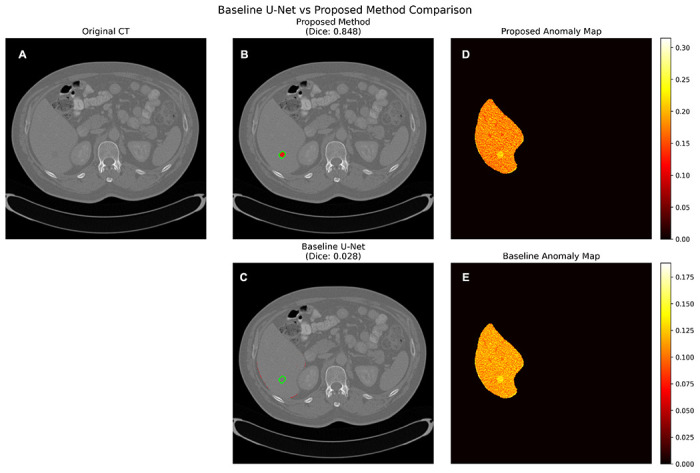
Baseline U-Net comparison. Side-by-side qualitative comparison between the proposed encoder–decoder difference model and a U-Net autoencoder baseline on the same slice. Each row shows the original CT (panel A), thresholded prediction overlay (using proposed method in panel B and baseline U-Net in panel C), and anomaly map within the liver mask (using proposed method in panel D and baseline U-Net in panel E). Model predictions are shown in red, and contours, shown in green, highlight true tumor boundaries. The U-Net baseline struggles with diffuse false positives, resulting in a lower Dice.
